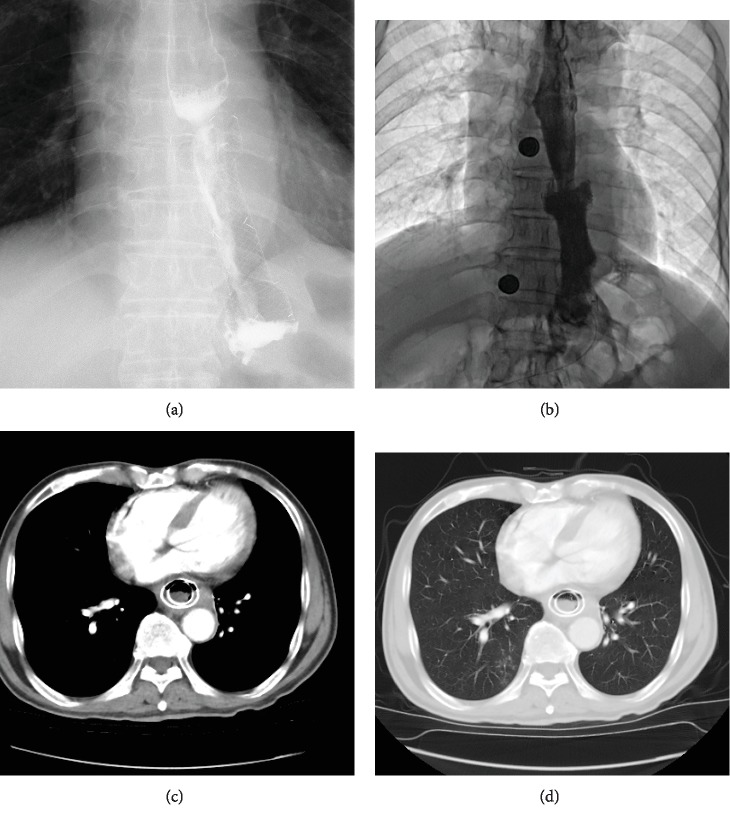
Additional stent placement for restenosis of stent (case 12). (a) Esophagography showed severe restenosis in the proximal end of stent 5 months later. (b) Another covered stent was inserted to relief restenosis. (c, d) A chest CT scan showed well expansion of stent and relief of restenosis.
